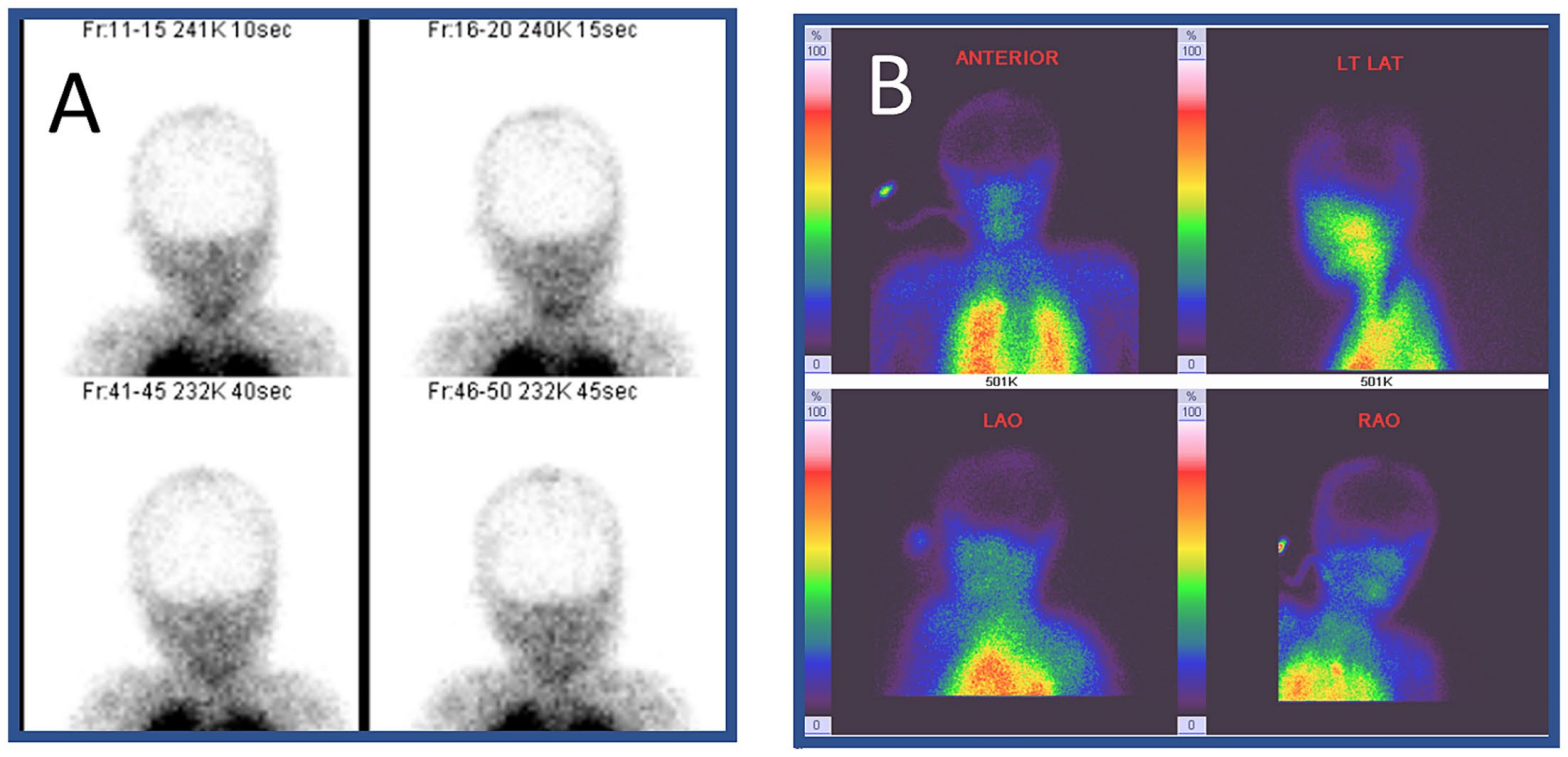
Radionuclide imaging of brain death. This 9-year-old girl suffered from severe pneumonia, requiring prolonged ventilation and extracorporeal membrane oxygenation. However, her condition continued to deteriorate. No brainstem reflexes were observed on day 7. She underwent single photon emission computed tomography (SPECT) with ^99^Technitium HMPAO. No radionuclide uptake (metabolic perfusion) was noted in the brain (hollow-skull sign) in the images in panels (A,B). (B) Shows increased radionuclide uptake in the extracranial structures including the nose area (hot nose sign).
